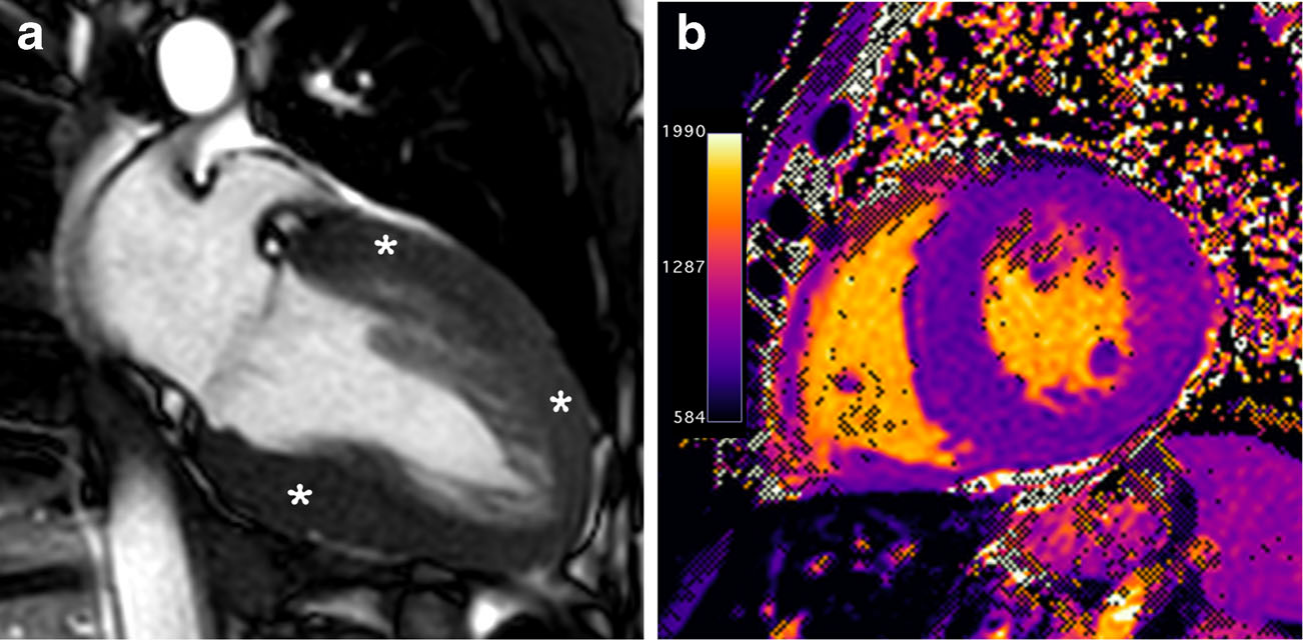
45-year-old man with gene-positive Anderson-Fabry disease. a, End-diastolic fouchamber SSFP cine MR image shows diffuse and symmetric LV wall hypertrophy (asterisks). b, Non-enhanced short-axis MOLLI native T1 map at 1.5 T shows significant shortening of T1 values (840 ms) in the hypertrophy segments. Post-gadolinium images were not obtained by renal impairment
